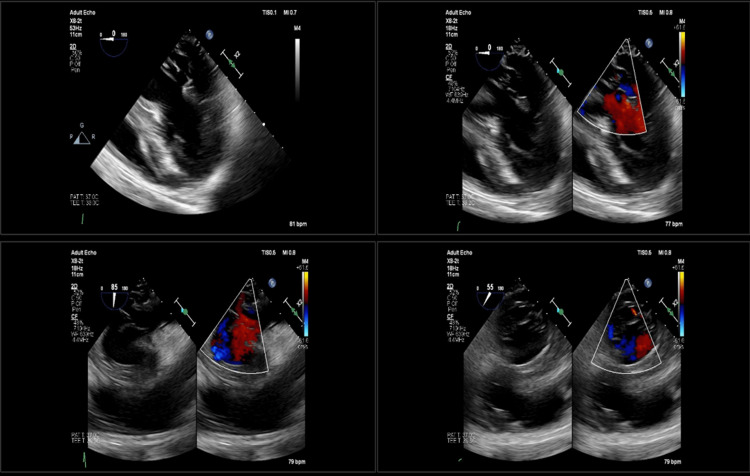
Transesophageal echocardiogram obtained during the first hospitalization without evidence of vegetation or intracardiac thrombus
